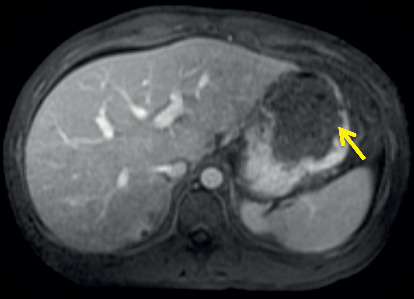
Magnetic resonance imaging of abdomen with gadolinium revealing a heterogeneous lesion in upper pole of the spleen measuring approximately 5.2 × 4.3 cm in size.
